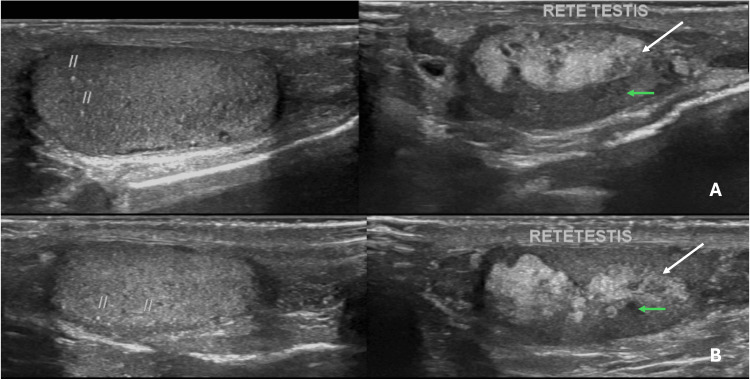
Bilateral scrotal ultrasound demonstrating imaging features of Stage III TARTs. (A) Left testis showing reduced volume with coarse rete testis calcifications (white arrow) and multiple intratesticular microliths (//), with a hypoechoic lesion adjacent to the mediastinum testis (green arrow). (B) Right testis showing similar findings of reduced testicular volume, coarse calcifications (white arrow), intratesticular microliths (//), and a hypoechoic mediastinal lesion (green arrow). Findings are consistent with Stage III disease. TART: testicular adrenal rest tumour
